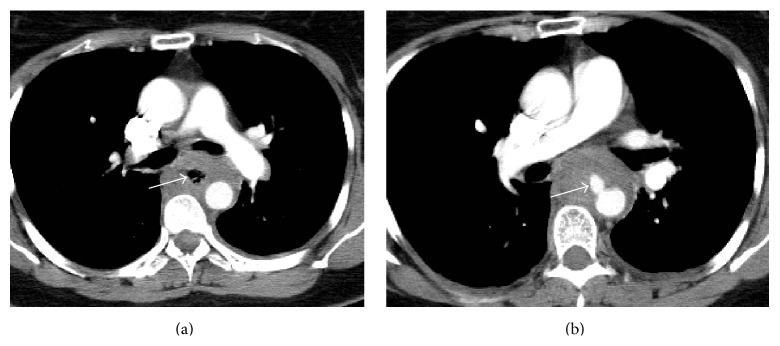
(a) Axial enhanced CT scan. Mediastinal collection with air bubbles within it (white arrow). (b) Axial contrast enhanced CT scan. Pseudoaneurysm of the thoracic aorta (white arrow).
